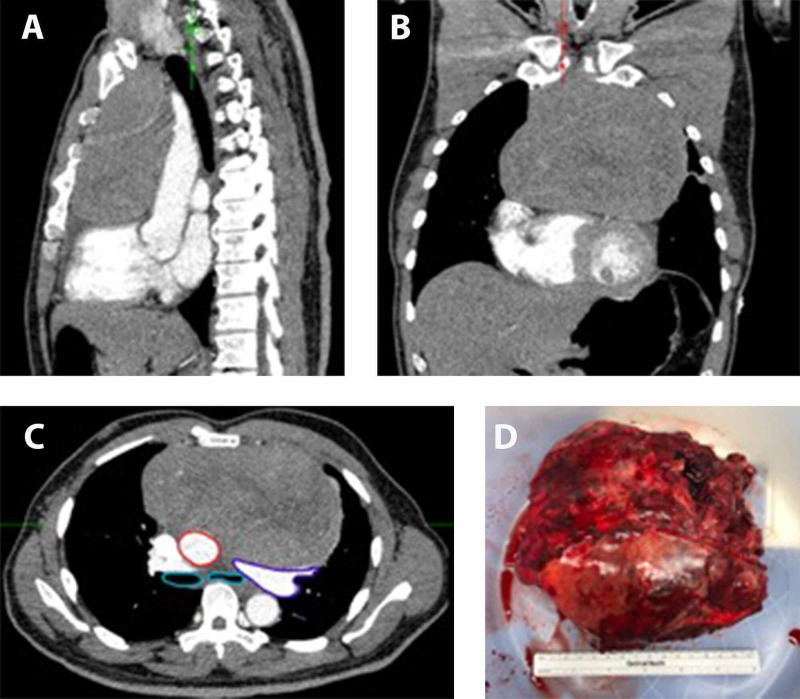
Radiographic and gross image of the mass Computed tomography imaging of the chest in (A) sagittal, (B) coronal, and (C) axial planes highlighting the extent of the mass. Panel C also highlights the extent of airway and vascular compression. The teal-colored circles reflect the bronchi and highlight left-sided bronchial compression. The structures outlined in red and purple represent the aorta and pulmonary artery, respectively, and the imaging highlights posterior displacement secondary to mass effect. (D) Photograph of the 16.1 cm × 9.2 cm × 12.3-cm mass after resection.
